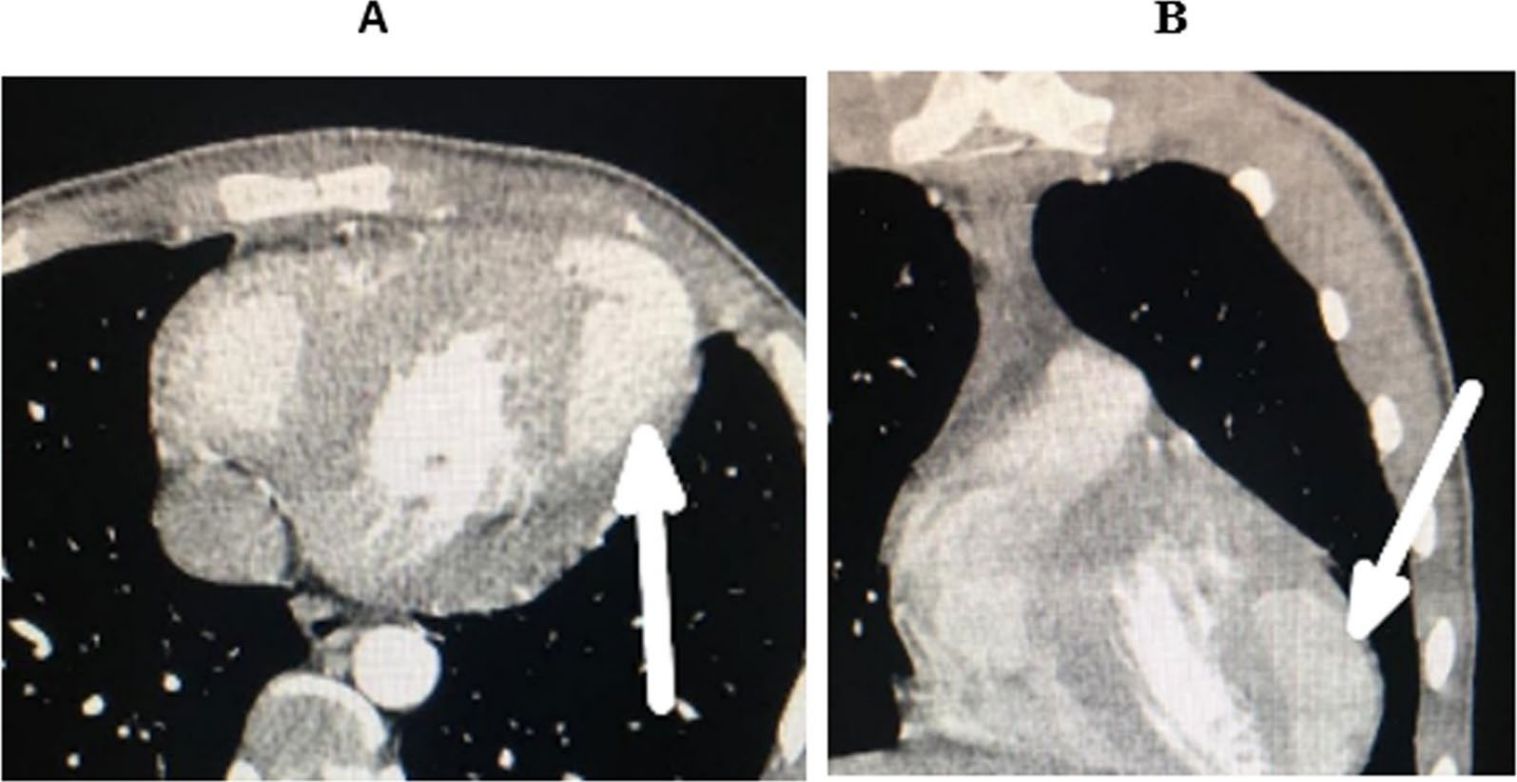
Computed tomography angiography of the chest showing the pseudoaneurysm of the left ventricular free wall (arrow). a axial view. b coronal view
